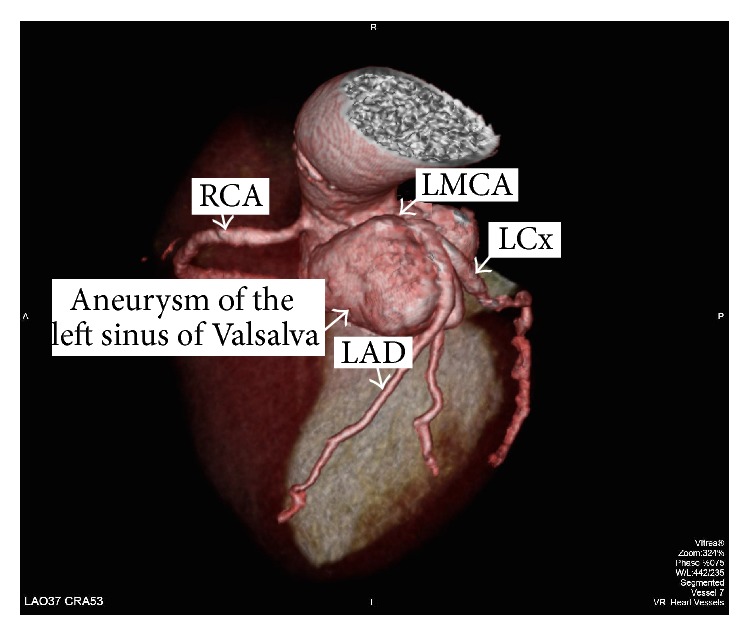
Post-PCI computed tomography angiography showing the giant aneurysm involving the three sinuses of Valsalva and its relationship with the major epicardial coronary arteries. PCI, percutaneous coronary intervention; RCA, right coronary artery; LMCA, left main coronary artery; LAD, left anterior descending; LCx, left circumflex.
